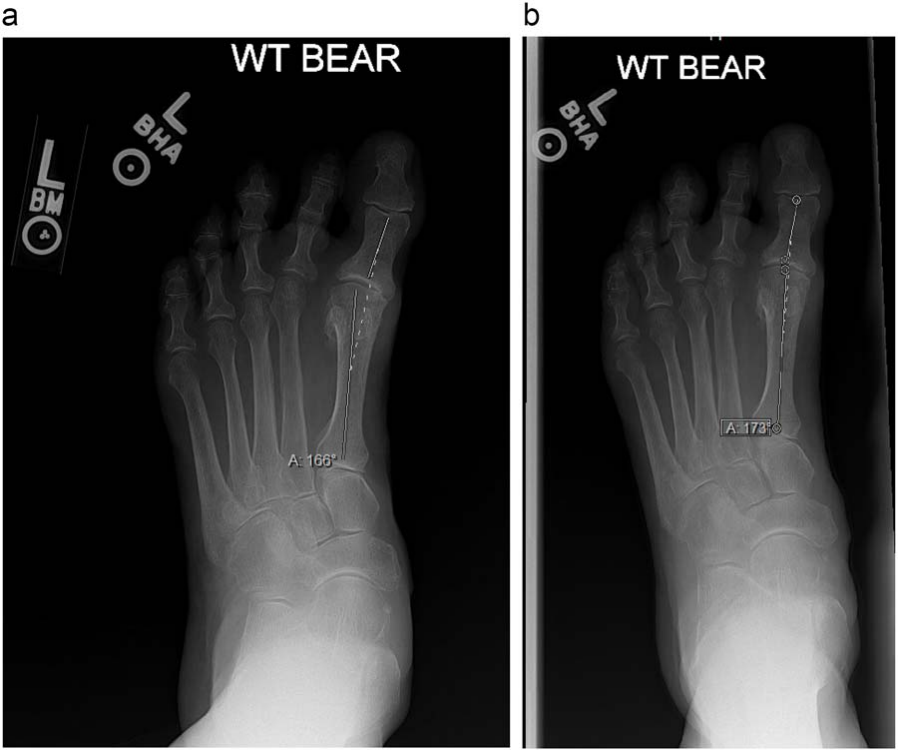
(a) and (b) are weight-bearing radiographs with angle measurements of the amount of hallux varus at the first MTP joint.
